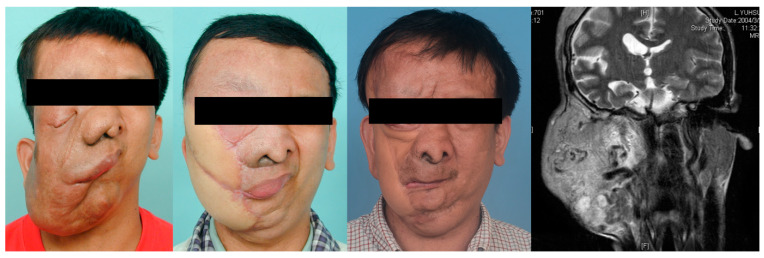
Clinical images of a 30-year-old man with hemifacial plexiform neurofibromatosis exhibiting no ipsilateral facial nerve function and vision. The patient was followed up by the multidisciplinary team for 12 years. Magnetic resonance imaging shows the extent of the plexiform tumor before surgical excision. Two sections of embolization of branches of the right external carotid artery, internal maxillary artery, and superficial temporal artery were completed before radical surgical excision. The distal right external carotid artery was ligated, and the tumor was extensively excised through scalp, preauricular, and submandibular incisions. Microsurgical free flaps (anterior lateral thigh flap and myocutaneous gracilis free flap) were transferred to reconstruct the facial defect. Additional procedures included orbitotomy, tumor resection (nasal and upper lip regions), labial suspension, canthopexies, eyelid reconstruction, and ocular prosthesis placement.
